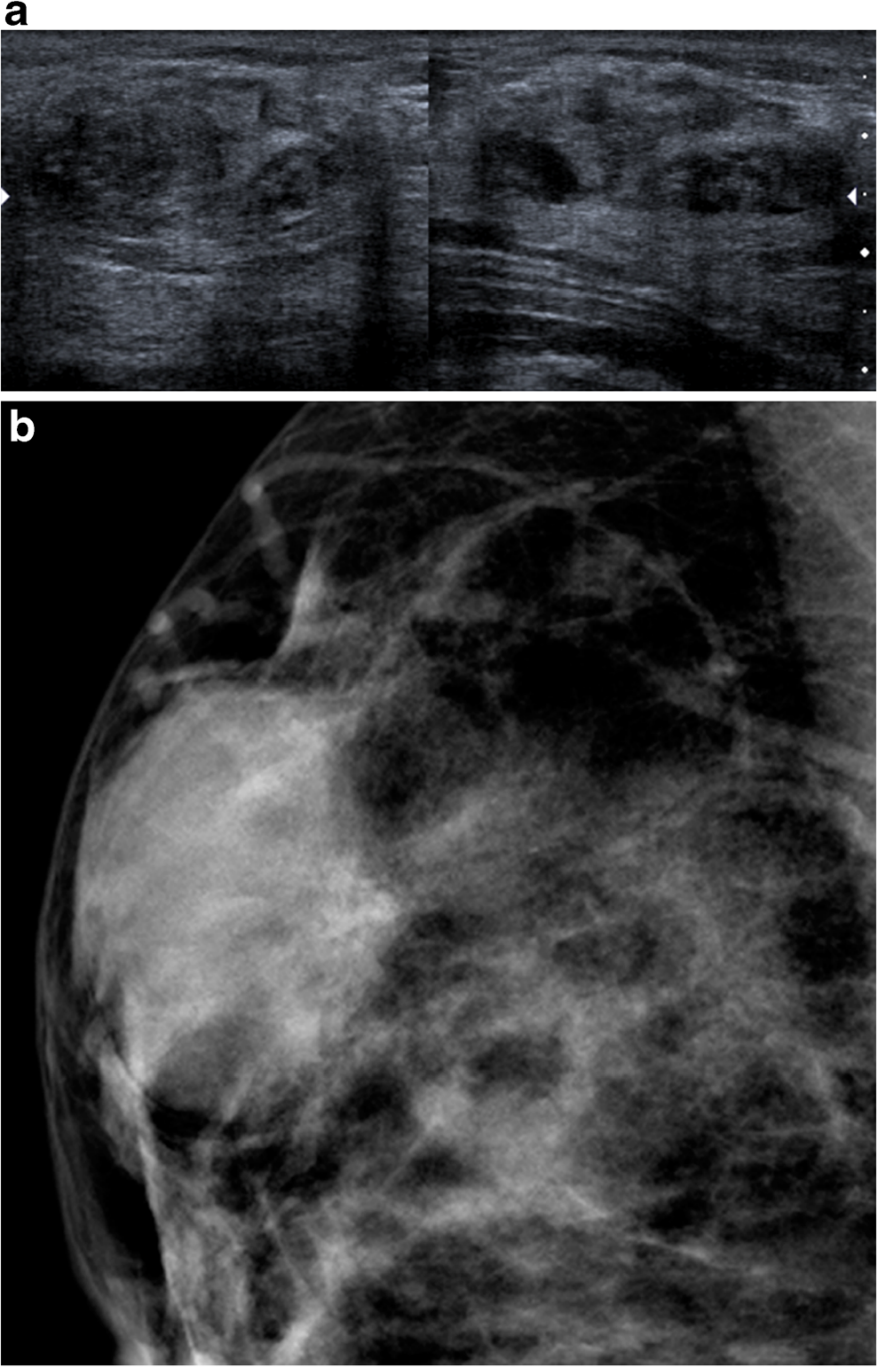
Hamartoma. a Ultrasound showing an oval-shaped mass with mixed echogenicity, globally isoechoic, with an edge effect artefact. b Mammography showing a well-circumscribed, dense, oval-shaped mass, with a slightly heterogeneous appearance related to its mixed content (fat and stroma)
